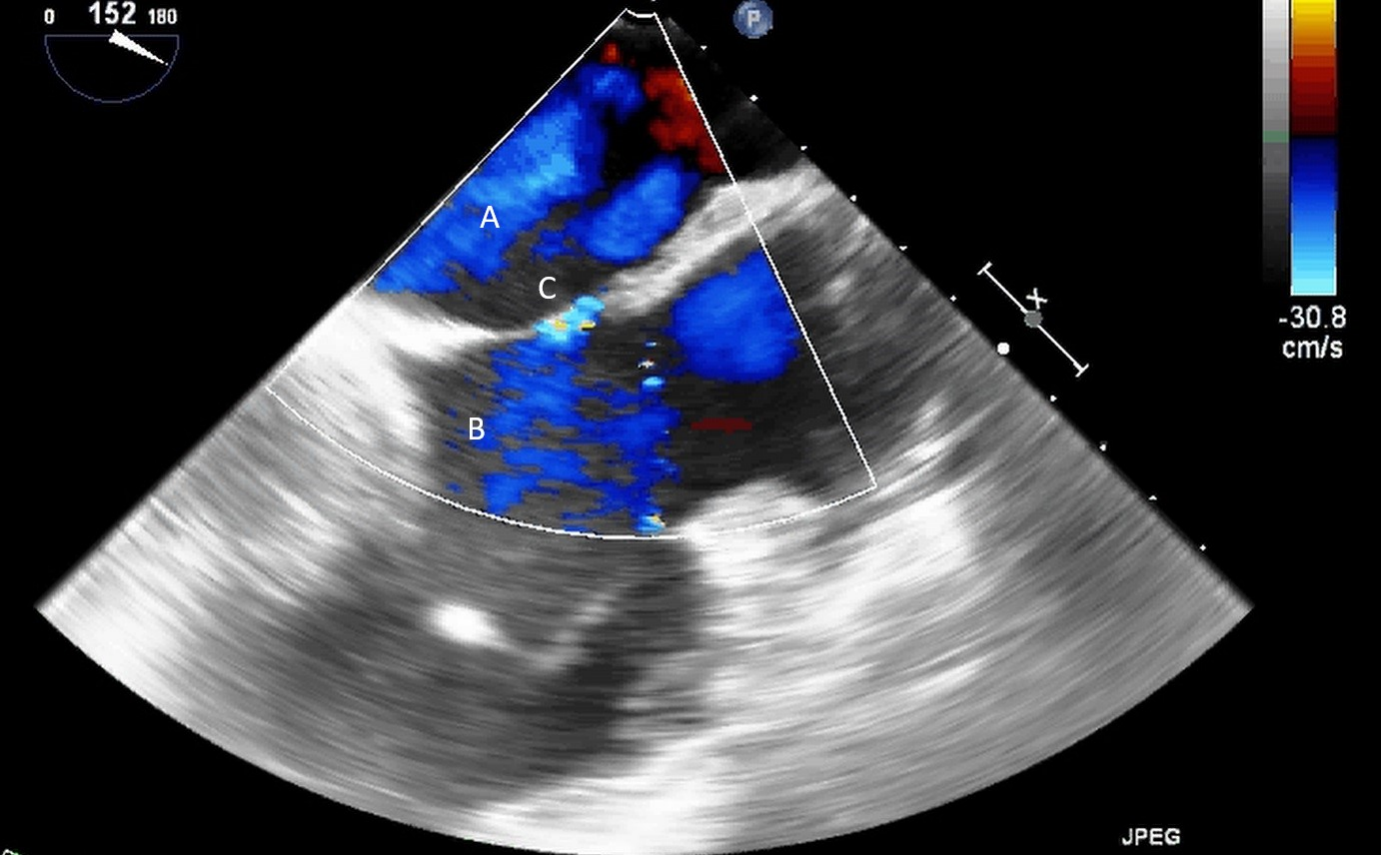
Transesophageal bi-caval view (Pre-LVAD implantation)
